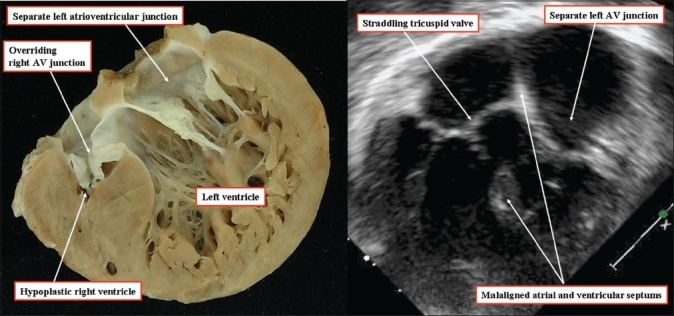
The anatomic section (left hand panel), and the echocardiogram (right hand panel) come from different patients. In both instances they show that the essence of straddling of the tricuspid valve is not in the malalignment between the atrial septum and the muscular ventricular septum, but in the setting of separate right and left atrioventricular junctions
